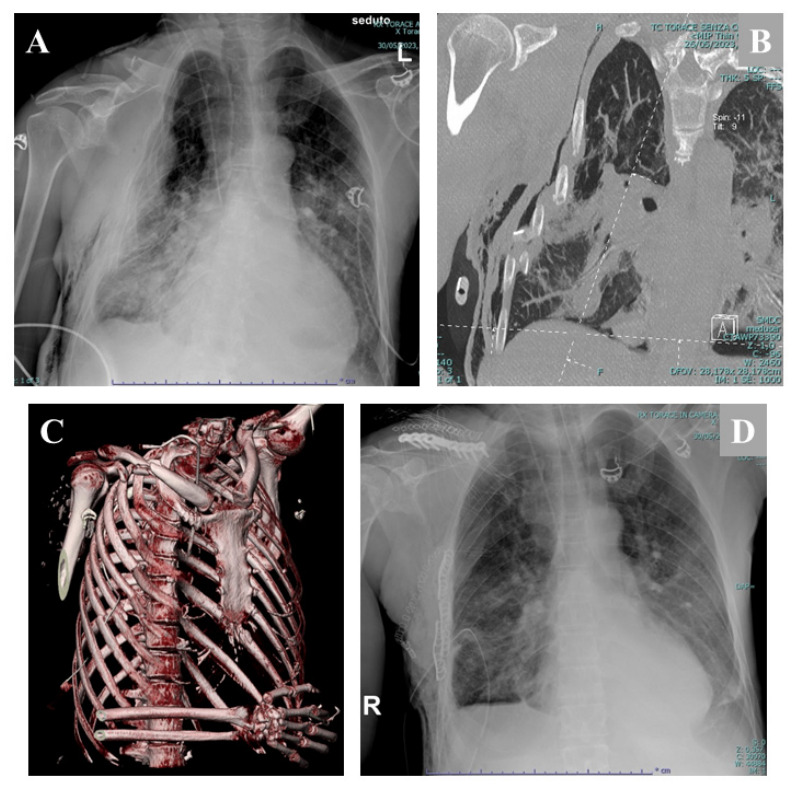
(A) Chest X-ray (AP projection): Multiple displaced rib fractures involving the right hemithorax are observed, with areas of pulmonary parenchymal contusion. A right-sided pneumothorax is present, with associated subcutaneous emphysema of the right chest wall. A displaced fracture of the mid-shaft of the right clavicle is also noted. (B) Chest CT (2D Coronal MIP reconstruction): In the same patient, the CT image demonstrates intrathoracic displacement of multiple right rib fracture fragments. Extensive subcutaneous emphysema is visible along the right chest wall. (C) Chest CT (3D reconstruction): The 3D CT image clearly demonstrates complex bifocal rib fractures involving the third to seventh ribs in the anterolateral region of the right hemicostat. (D) Postoperative chest X-ray (AP projection): Status post-surgical stabilization of right rib fractures with locking rib plates. The rib fracture fragments appear well aligned. There is near-complete resolution of the previously noted pulmonary contusion and of the subcutaneous emphysema of the chest wall.
